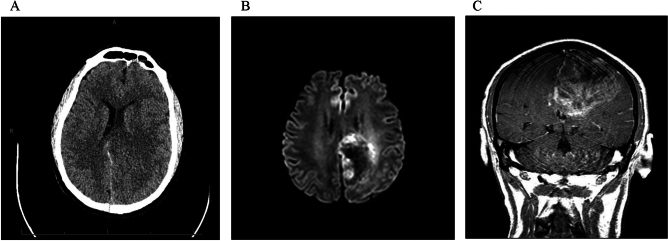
Day 2 brain imaging. A Axial CT brain without contrast demonstrating interval worsening of the left paramedian parieto-occipital hypoattenuation with more severe mass effect and rightward midline shift. B Axial DWI MRI with plus without contrast demonstrating left parietal lobe lesion with involvement of the splenium of the corpus callosum with a leading edge of diffusion restriction. C Coronal MRI post-gadolinium contrast T1-weighted image depicting patchy enhancement involving the left parietal lobe and midline shift
